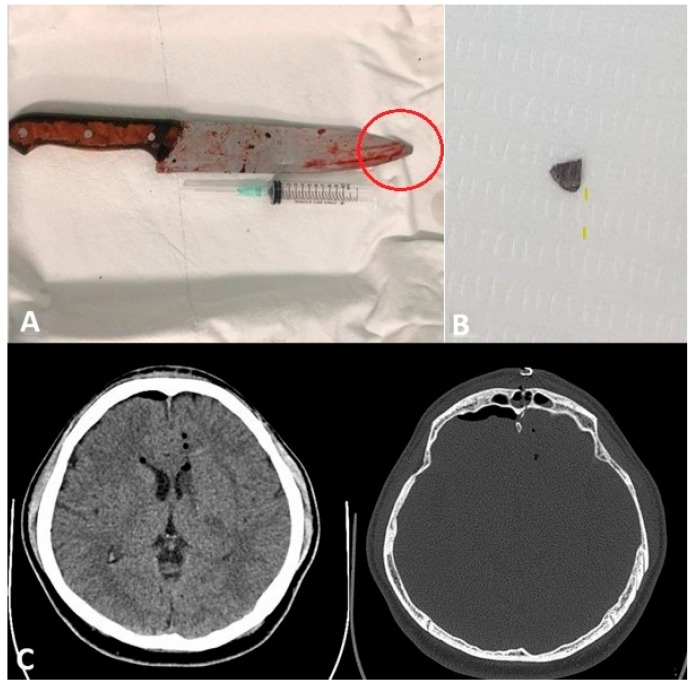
Intraoperative and postoperative outcomes. (A) Photograph of the extracted kitchen knife (shown with a 10 cc syringe for scale). Note the blunted tip suggesting fragmentation (red circle). (B) The recovered metallic tip fragment (approximately 7 mm in length) which was found embedded near the inner wall of the frontal sinus and successfully removed. (C) Postoperative axial CT scan showing total removal of the foreign body and cranialization of the frontal sinus.
